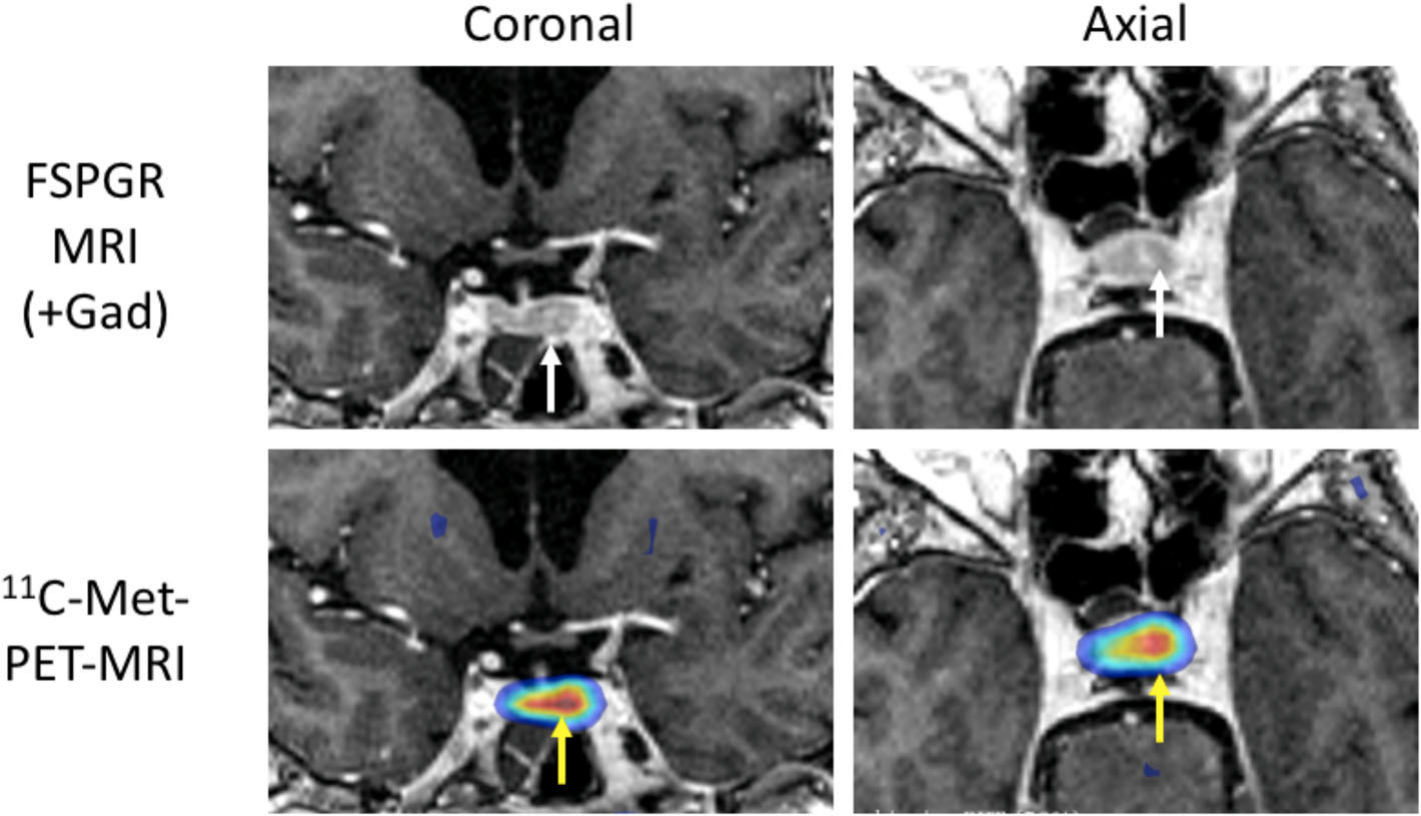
^11^C-methionine PET/CT coregistered with volumetric MRI. ^11^C-methionine PET/CT coregistered with volumetric MRI Cornonal and axial views showing a focus of increased tracer uptake in the left side of the sella (yellow arrows) corresponding to the site of a possible microadenoma on MRI (white arrows)
